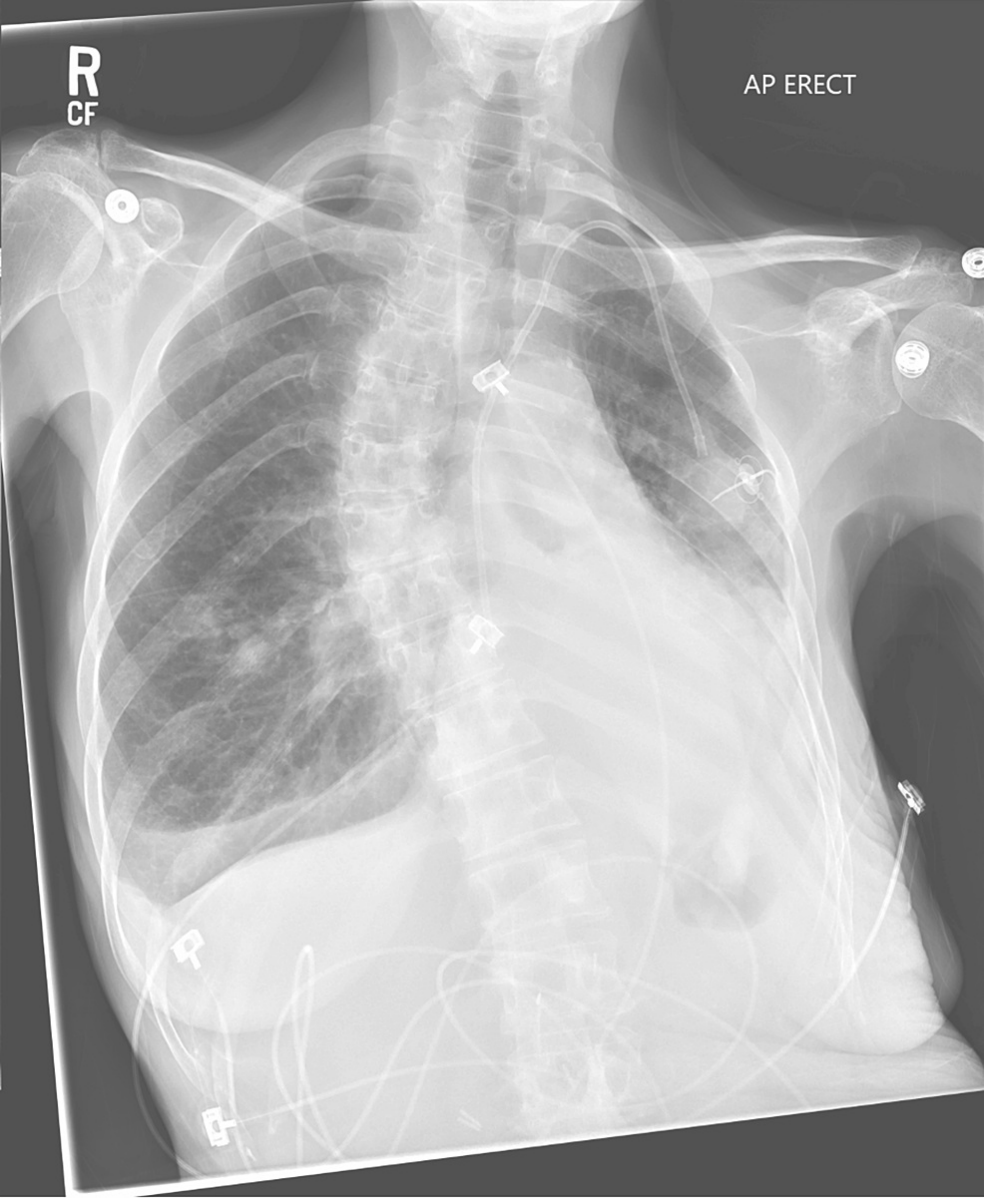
Anteroposterior CXR showing persistent left lower lobe atelectasis and right pleural effusion from March 2023. CXR: chest X-ray
